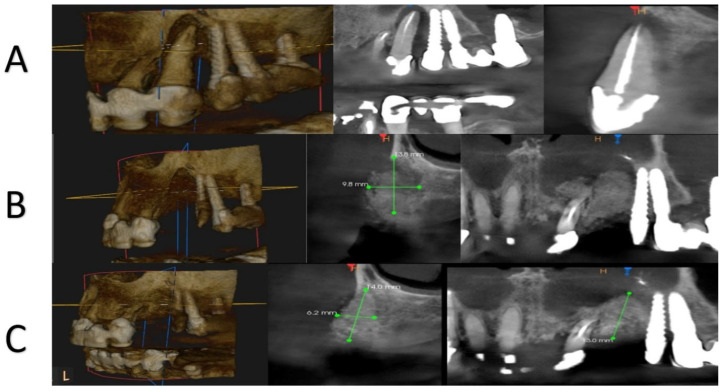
CBCT panel showing (A)—preoperative planning of extraction; (B)—immediate post-operative situation after socket filling with SB; (C)—condition four months after regeneration using SB.
